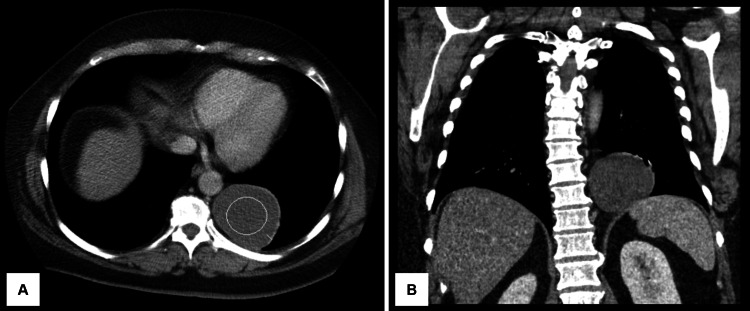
CT scan of the thorax A low-density mass (circled) is present in the left aspect of the posterior mediastinum, shown on the axial plane (A) and the coronal plane (B) of a CT scan of the chest. CT: computed tomography.
